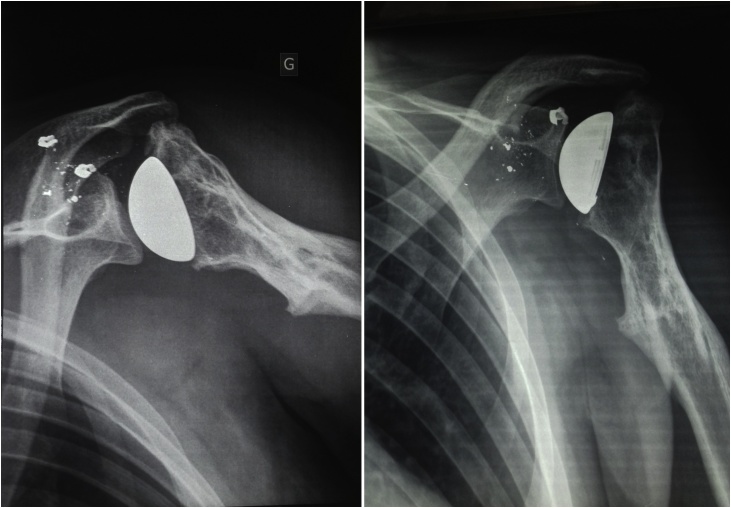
Two months radiographs showing a well positioned implant but a malunion of the greater tubercle.
